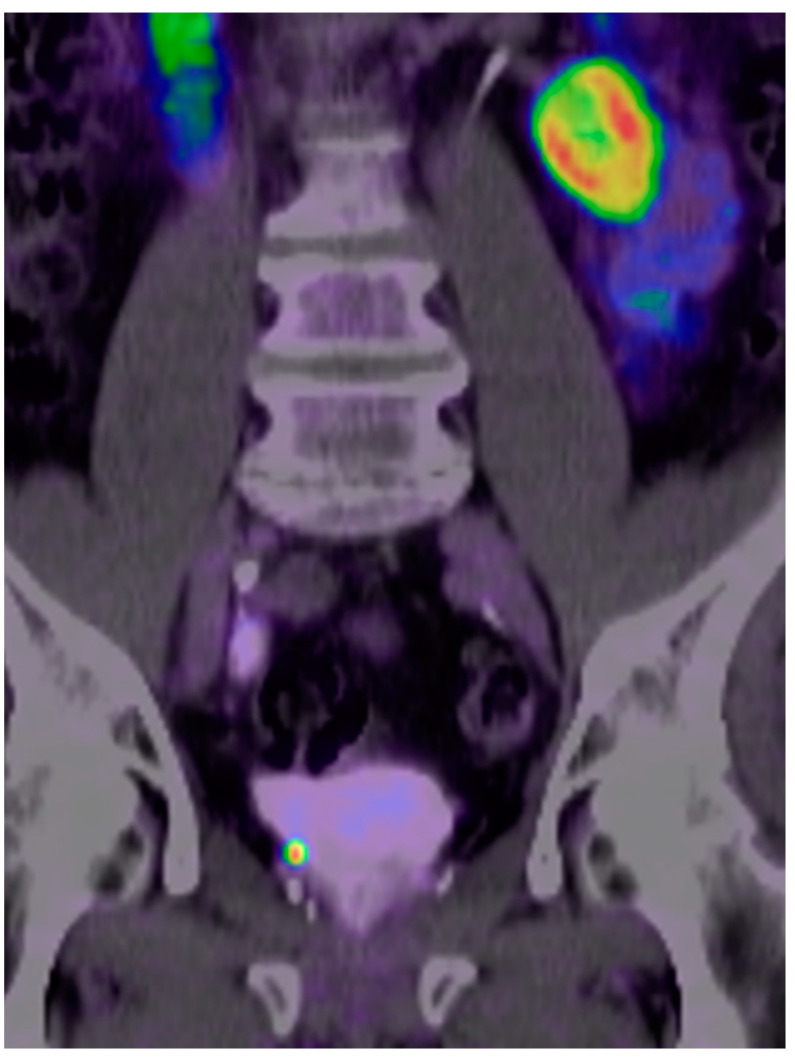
Case study of a prostate cancer (PC) patient who was pretreated with a radical prostatectomy, seminal vesicle removal, and lymphadenectomy (pT3a, pN0 [0/14], cM0, G3) in October 2020 with an initial prostate-specific antigen (PSA) of 6.77 ng/mL. Postoperatively incomplete PSA drop to 0.08 ng/mL. In April 2021 (time of PSMA imaging), the PSA level rose again to 0.197 ng/mL. [^18^F]PSMA-1007 positron-emission tomography/computed tomography (PET/CT) shows a local recurrent PC-lesion near the base on the right in the former seminal vesicle with high prostate-specific membrane antigen (PSMA)-avidity (maximum standardized uptake value/SUV_max_ of 24.3 in the routine images and of 32.4 in the late-stage images) as a correlate of the biochemical recurrence (BCR). (The imaging and data were collected from a Practice of Radiology and Nuclear Medicine in Cologne/Germany, named “Praxis im KölnTriangle”).
